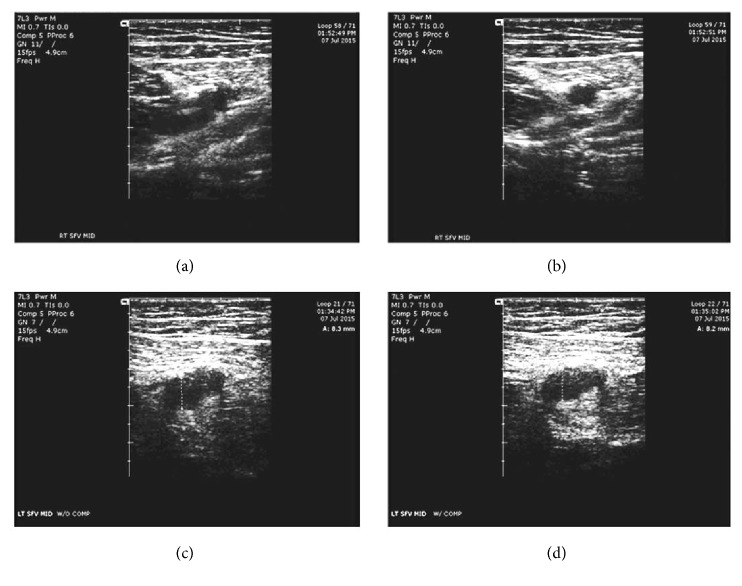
Lower extremity ultrasound findings upon initial evaluation. (a) Uncompressed right superficial femoral vein patent. (b) Compressed right superficial femoral vein completely collapsed. (c) Uncompressed left superficial femoral vein at 8.3 mm. (d) Compressed left superficial femoral vein at 8.2 mm.
